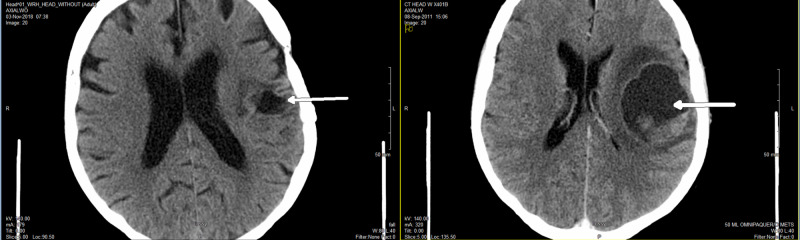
CT scan in November 2018 showing CR in brain (left) comparing to the original CT in 2011 (right) CR, complete response
